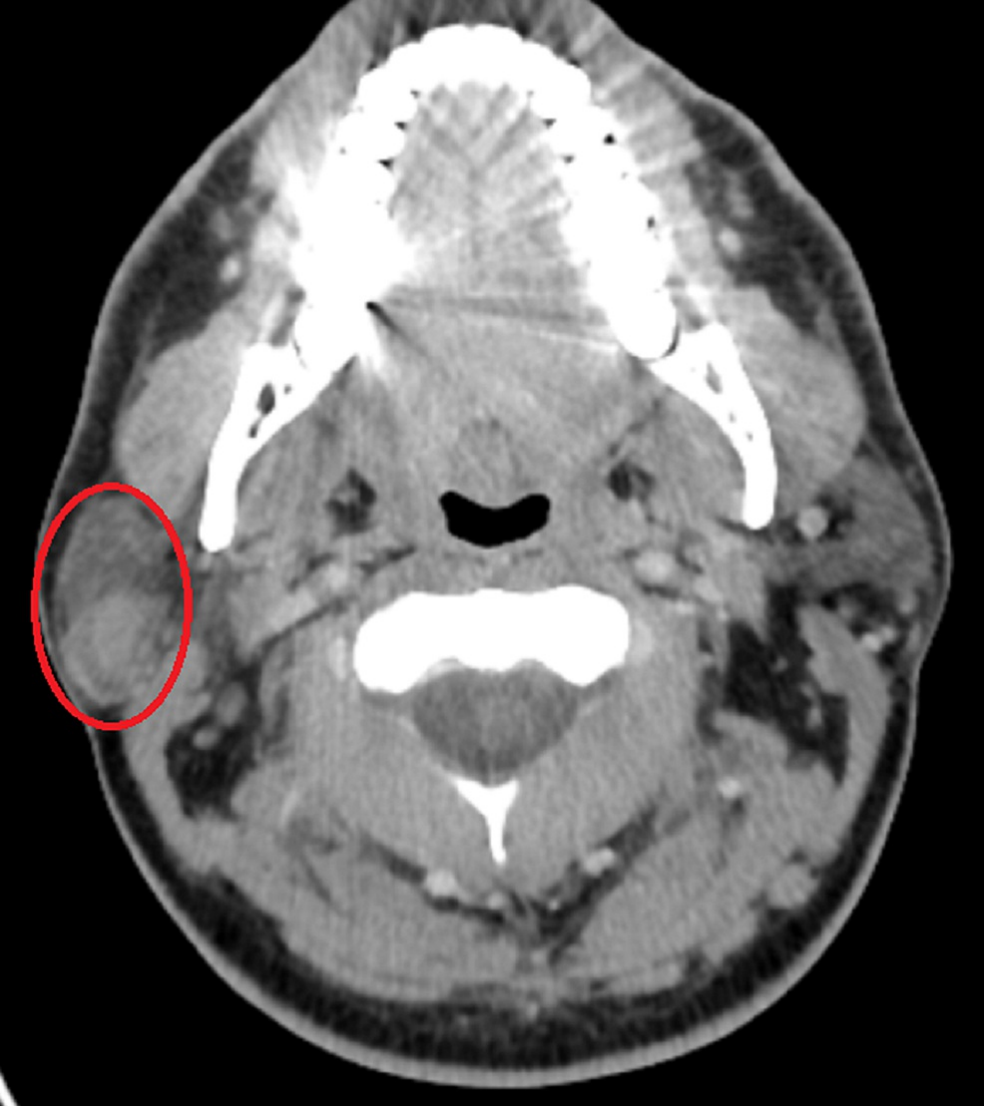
CT scan showing the tumor.
